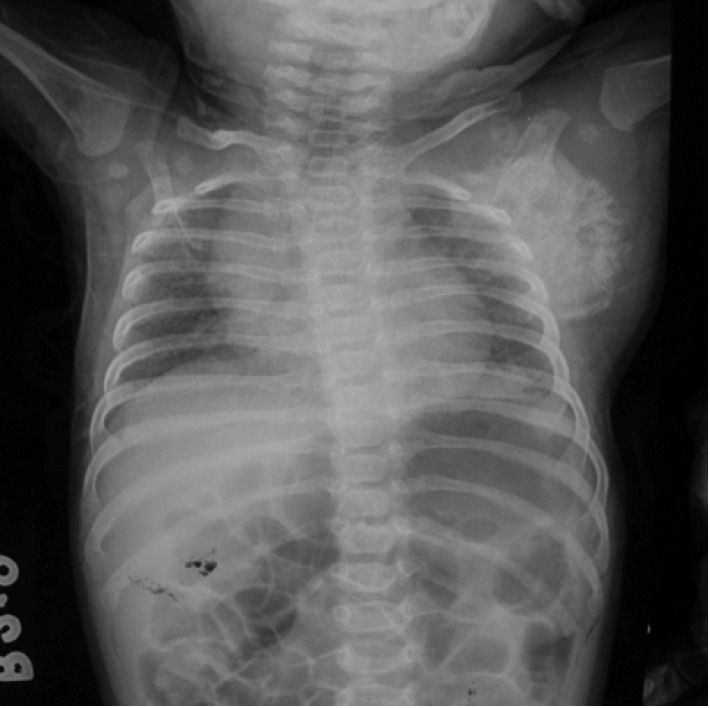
Anteroposterior radiograph of the right shoulder
